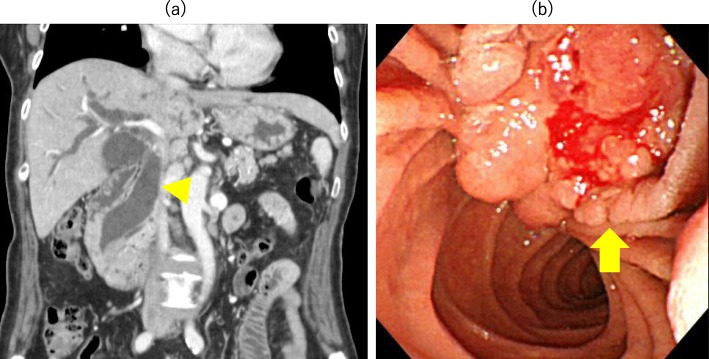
a Abdominal CT revealing a dilation of both the common bile duct (arrow head) and the main pancreatic duct. b Upper gastrointestinal endoscopy revealing a mass lesion (arrow) in the ampulla of Vater
